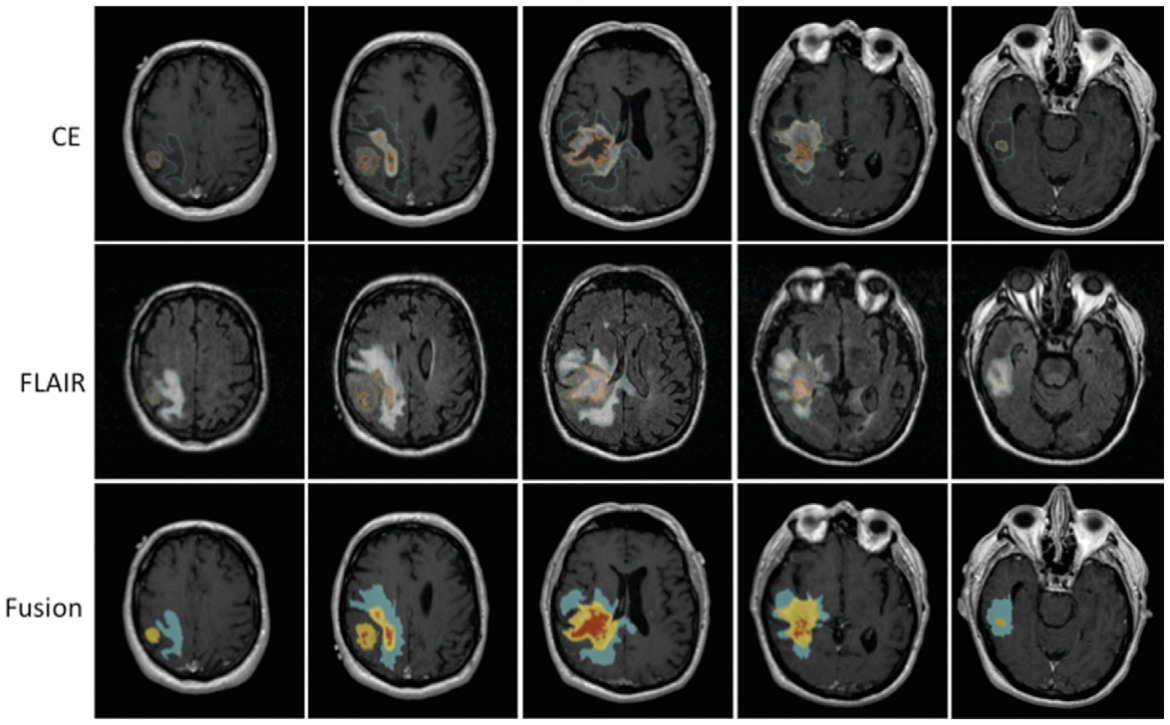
Low FLAIR patient. A 67 year old male patient with a right temporo-parieto-occipital GBM. The segmented edema/tumor infiltration (blue), enhancement (yellow) and necrosis (orange) are seen overlaid on a base post- contrast T1WI. These images demonstrate a patient with a GBM and a large amount of peritumoral FLAIR hyperintensity reflecting edema and tumor infiltration.
